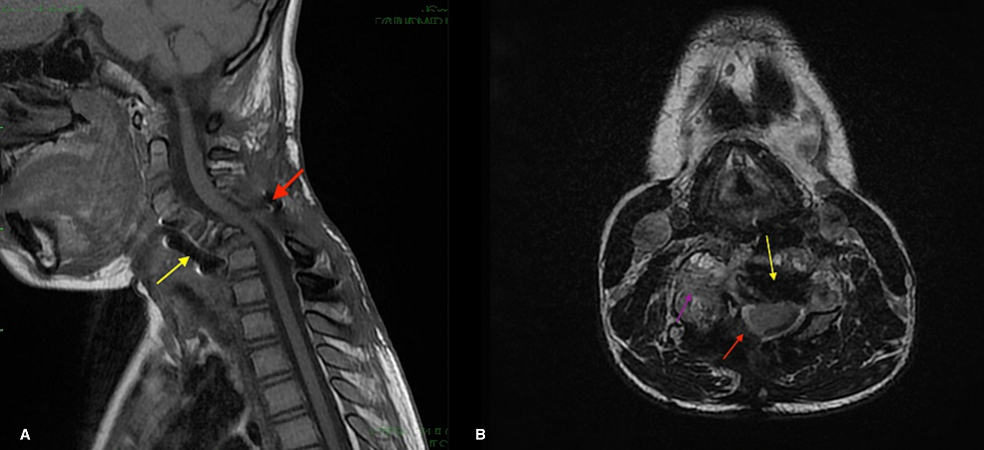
T1 sagittal (A) and T2 axial MRI at two years showing a stabilized lesion, spinal cord decompression (red arrow), a remnant around the vertebral artery (purple arrow), and anterior cervical plate (yellow arrow)
